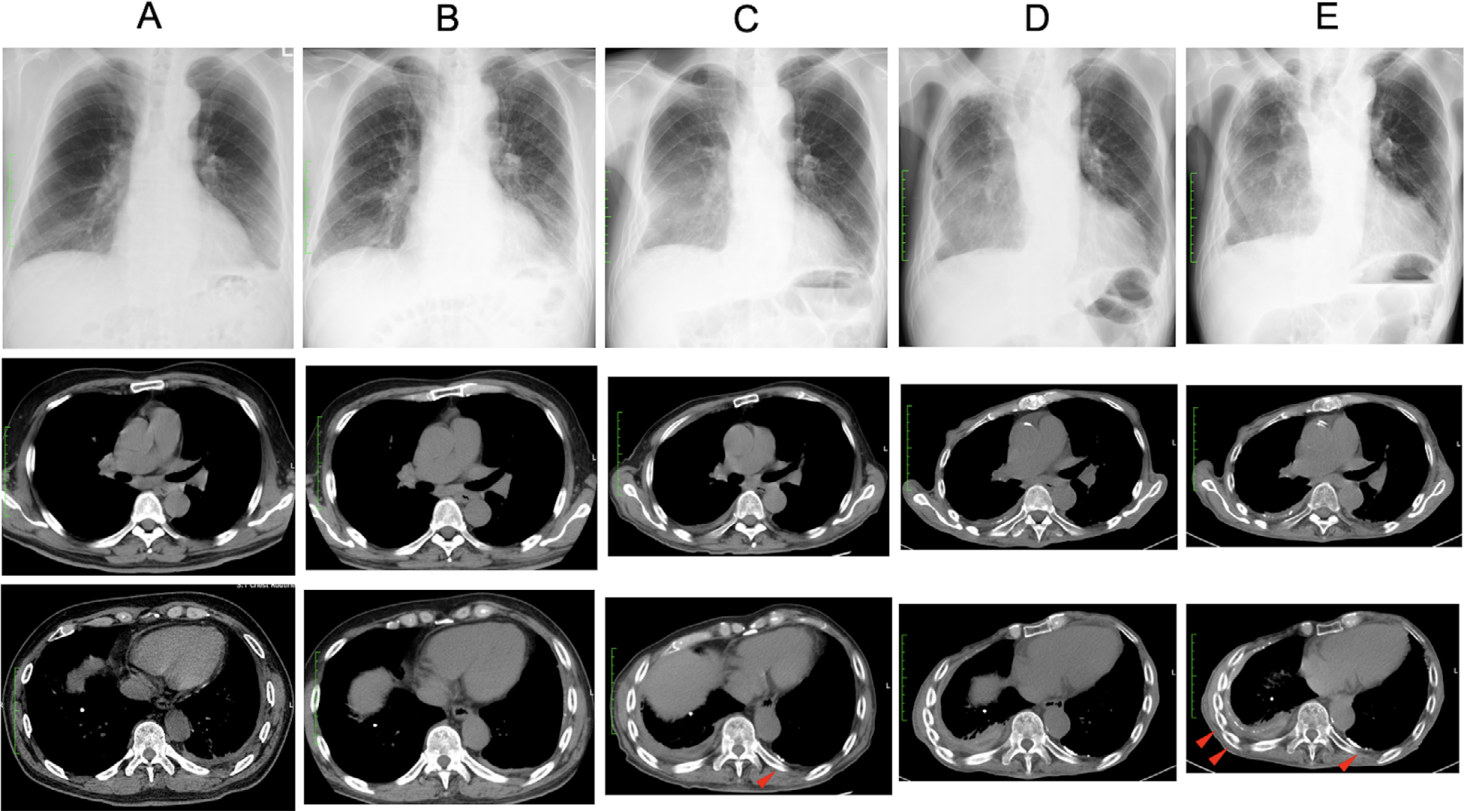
Chest X‐ray and chest computed tomography (CT) six years before (A), three years before (B), and on the day of the first visit (C). X‐ray: Costophrenic angle blunting is observed first on the left (A, B) and then on the right (C). CT: Left pleural effusion is replaced by pleural thickening with calcifications (C, arrow). Chest X‐ray and chest CT on the 44th (D) and 56th (E) months from the day of the first visit. X‐ray: The size of the right lung is declined gradually over 11 years (D, E). CT: Both right and left diffuse pleural thickening with calcifications show progression (E,arrows).
